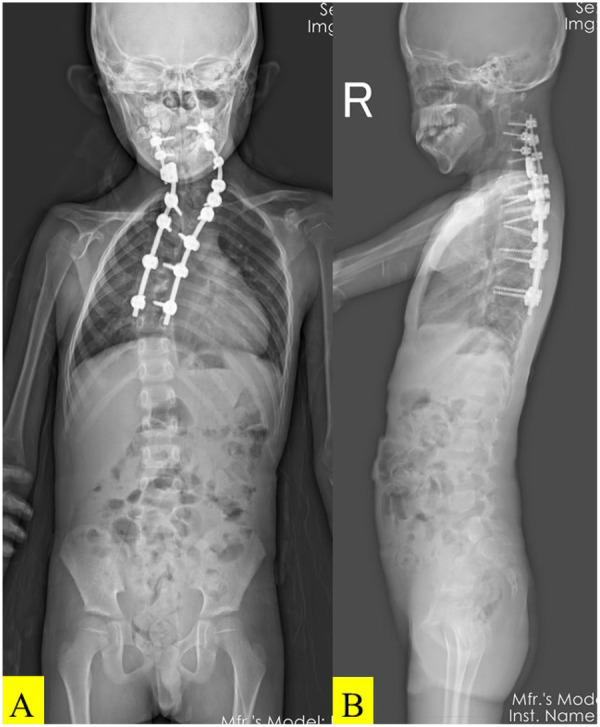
Postoperative standing anteroposterior and lateral X-ray images of the full-length spine. The Cobb angle of the cervical segment was 46°, and that of the thoracic segment was 40° (A, B).
